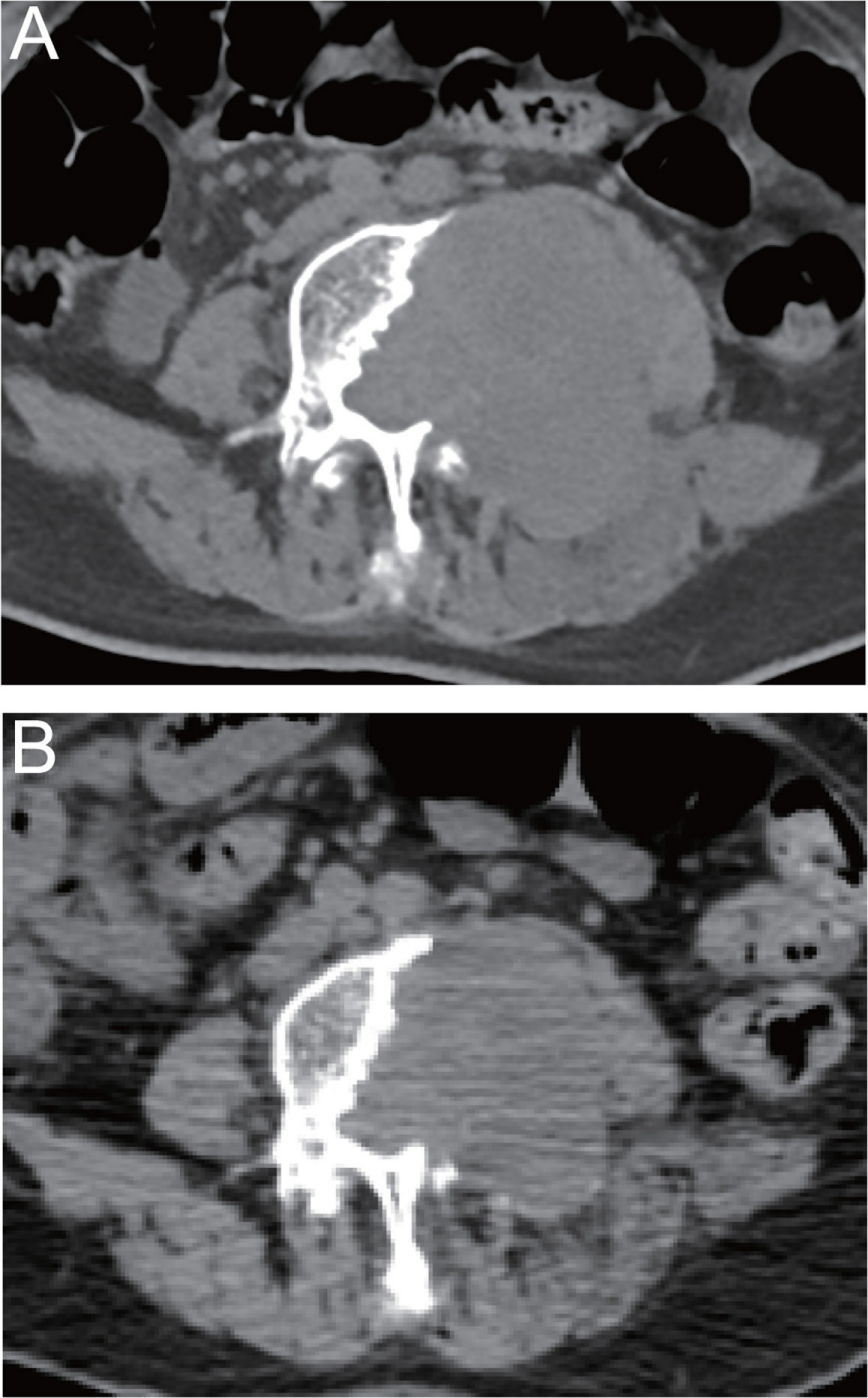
Regression of a bone metastatic radioiodine-refractory follicular thyroid cancer lesion in a 74-year-old male patient. Despite ^131^I-avidity demonstrated by post-therapeutic ^131^I scan, the metastatic lesion in fourth lumbar vertebra is enlarged one month after ^131^I administration (A), resulting in an inability to walk. Five months after the initiation of apatinib treatment, the patient could walk again, with the CT scan showing a 15.0% decrease in the largest diameter of the mass from baseline (B).
